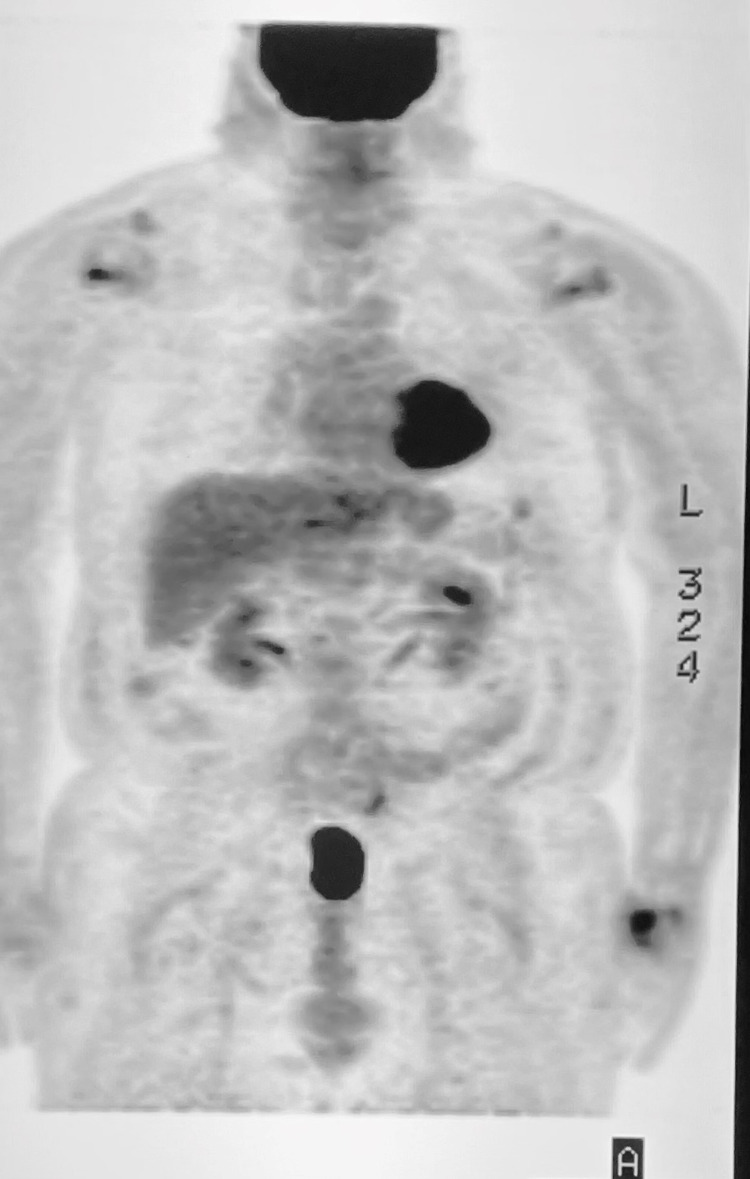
PET-CT six months post treatment with IV rituximab showing complete resolution of lesions.
